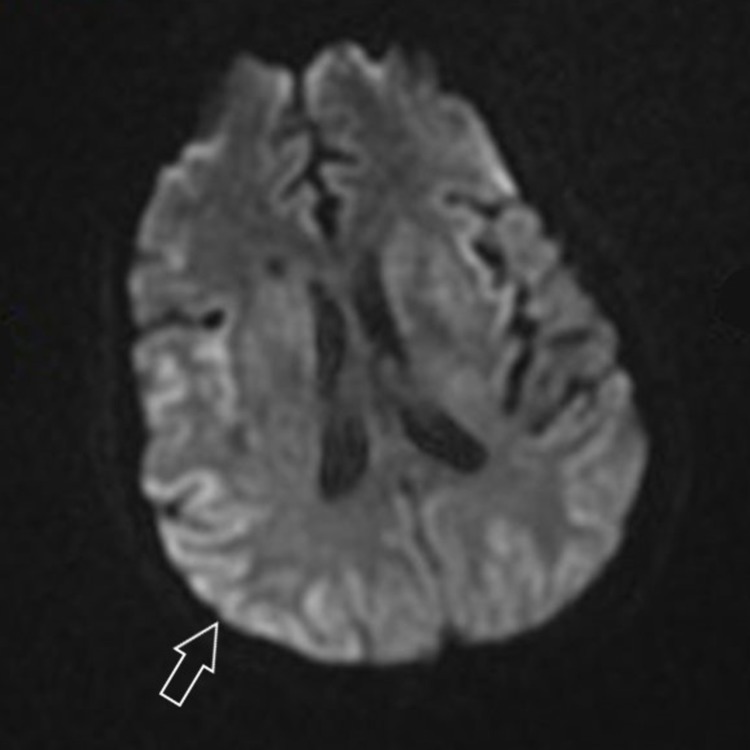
Magnetic Resonance Imaging of the brain showing reversal of diffusion weighted abnormalities Arrow over right parietal lobe showing areas of less hyperintensity denoting reversal of hypoxic injury
